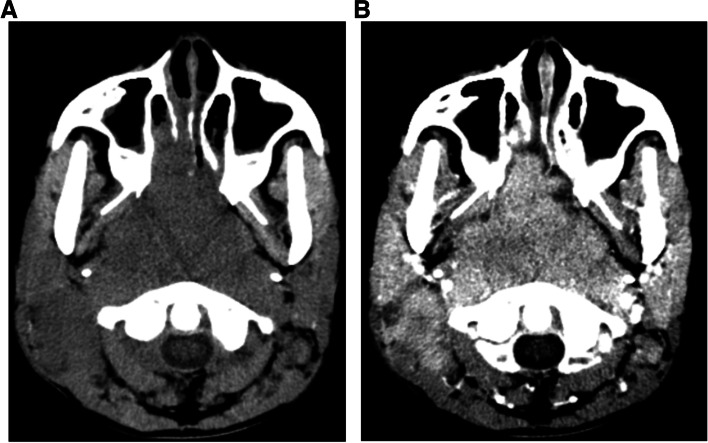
A An unenhanced axial CT at the level of the maxillary sinuses. There was a large iso-dense nasopharyngeal mass obliterating both torus tubarius and fossae of Rosen Muller. The mass was bound laterally by the medial pterygoid muscles and measured 5.4 cm × 6.0 cm. There were no calcifications seen with in the mass. B A contrast enhanced axial CT at the same level and demonstrated the same features as on A. In addition, there was mild homogeneous enhancement of the mass. This was a diagnosis of nasopharyngeal carcinoma, made on both A and B
